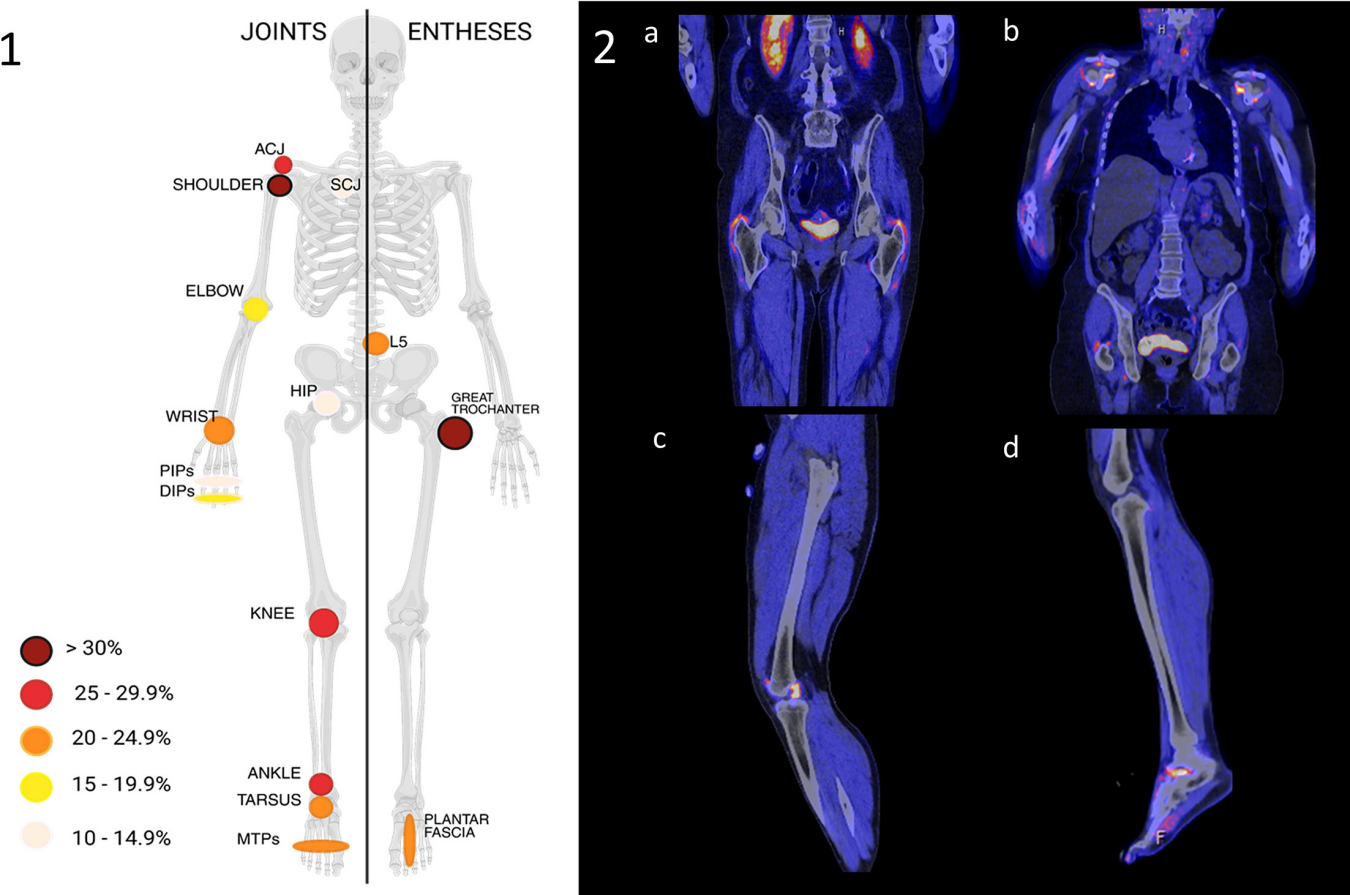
Anatomical distribution of ^68^Gallium-labelled fibroblast activation protein inhibitor (^68^Ga-FAPI) uptake. (1) Graphical representation of the 15 sites that most commonly showed increased fibroblast activation in patients with psoriasis. The colour scale reflects the site-specific frequency of ^68^Ga-FAPI uptake expressed as a percentage on the total of all patients: dark red: >30%; light red: 25–29.9%; orange: 20–24.9%; yellow: 15–19.9%; white: 10–14.9%. (2) PET/CT images of sites that most commonly show increased ^68^Ga-FAPI uptake: great trochanter (a), shoulder (b), knee (c) and ankle (d). ACJ, acromioclavicular joint; DIPs, distal interphalangeal joints; MTPs, metatarsophalangeal joints; PET/CT, positron emission tomography /computed tomography; PIPs, proximal interphalangeal joints; SCJ, sternoclavicular joint.
